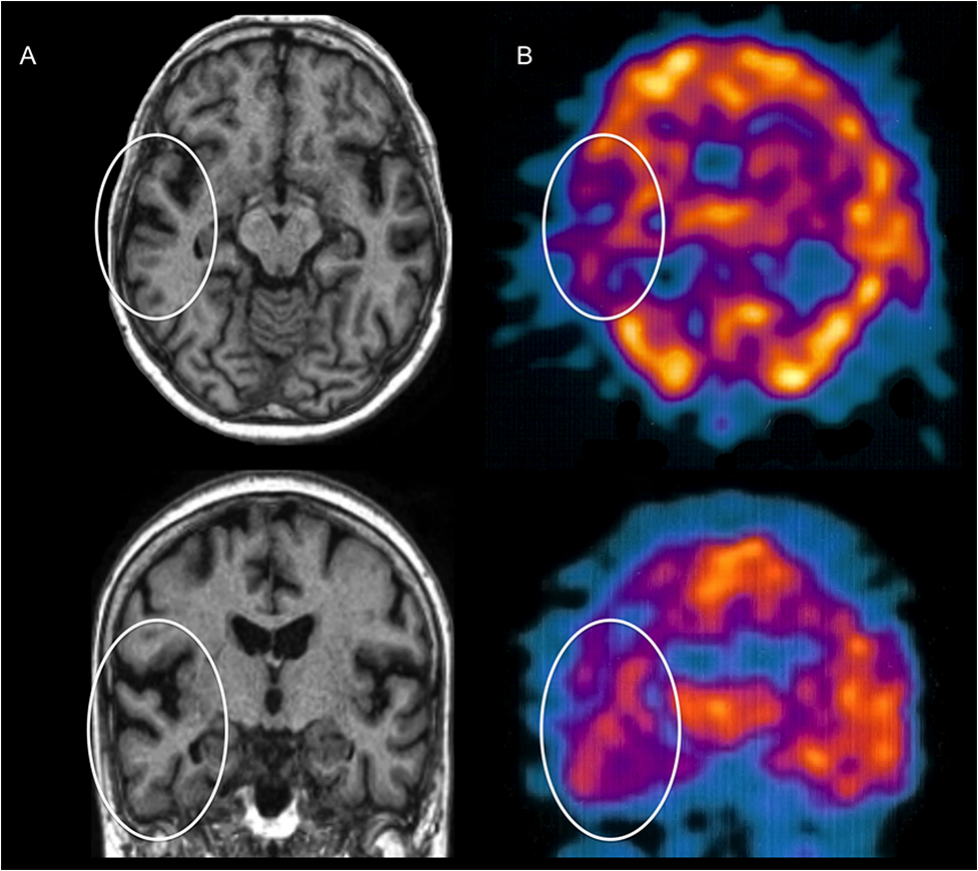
Anatomical MRI and SPECT of Patient’s GN Brain. (A) T1-weighted MRI transversal and coronal images showing atrophy in the left temporal lobe (white circles). (B) SPECT transversal and coronal images showing hypoperfusion in the left tempo-parietal region (white circles).
